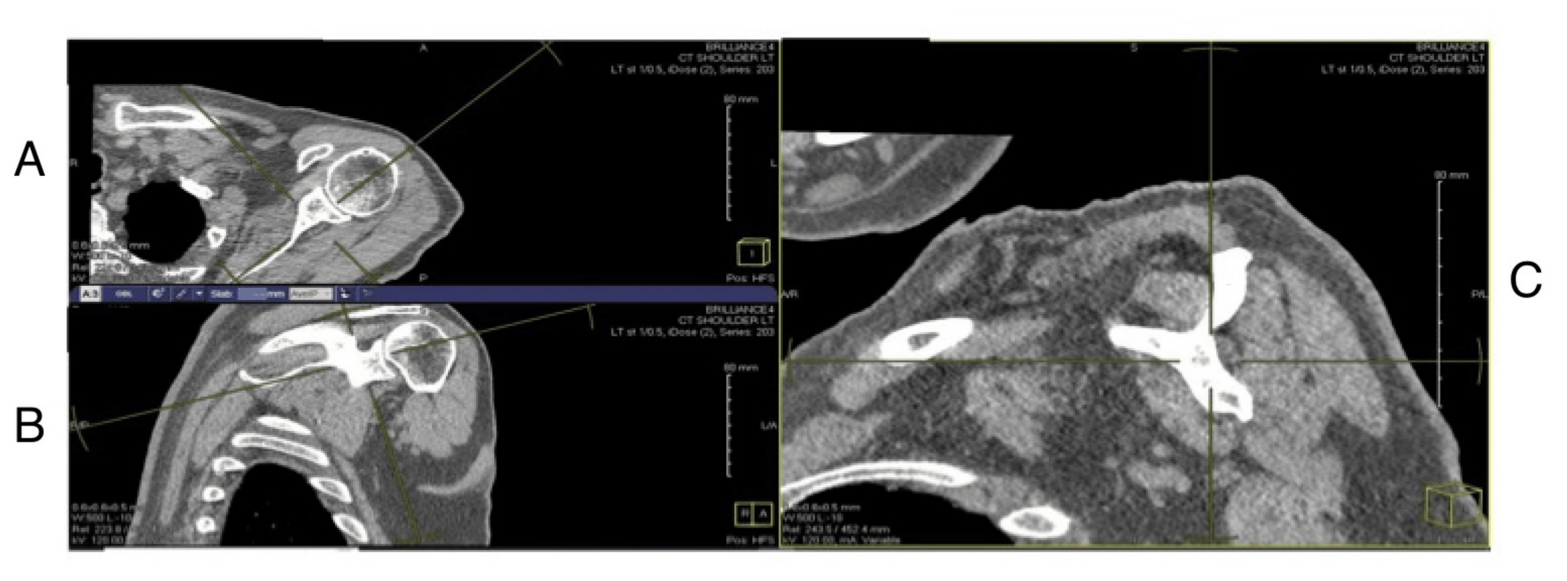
Creating the Y-view of the shoulder on CT images: (A) axial view, (B) coronal view, and (C) oblique sagittal view used to generate the Y-view.
